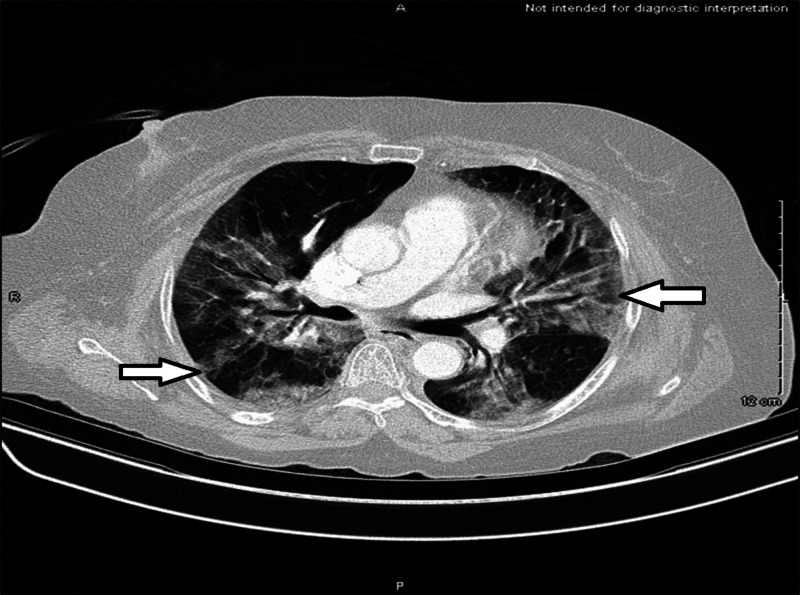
CT chest on hospital day 3 showing bilateral ground-glass opacities.
